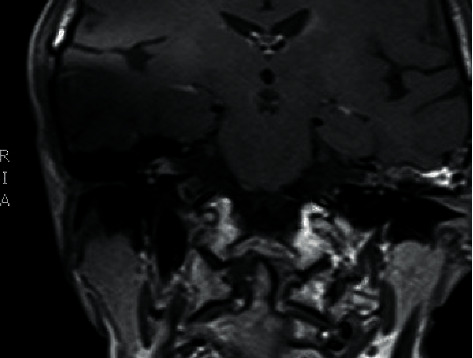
Coronal overview T1 MRI GAD of cerebellopontine angle 2 years after cochlear implantation of the right ear.
